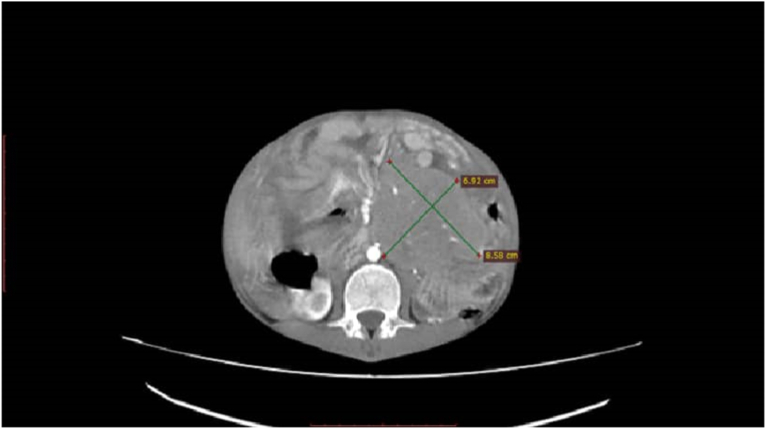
Full-body computed tomography (CT) scan demonstrating a poorly defined heterogeneous mass measuring approximately 85x70 mm.
